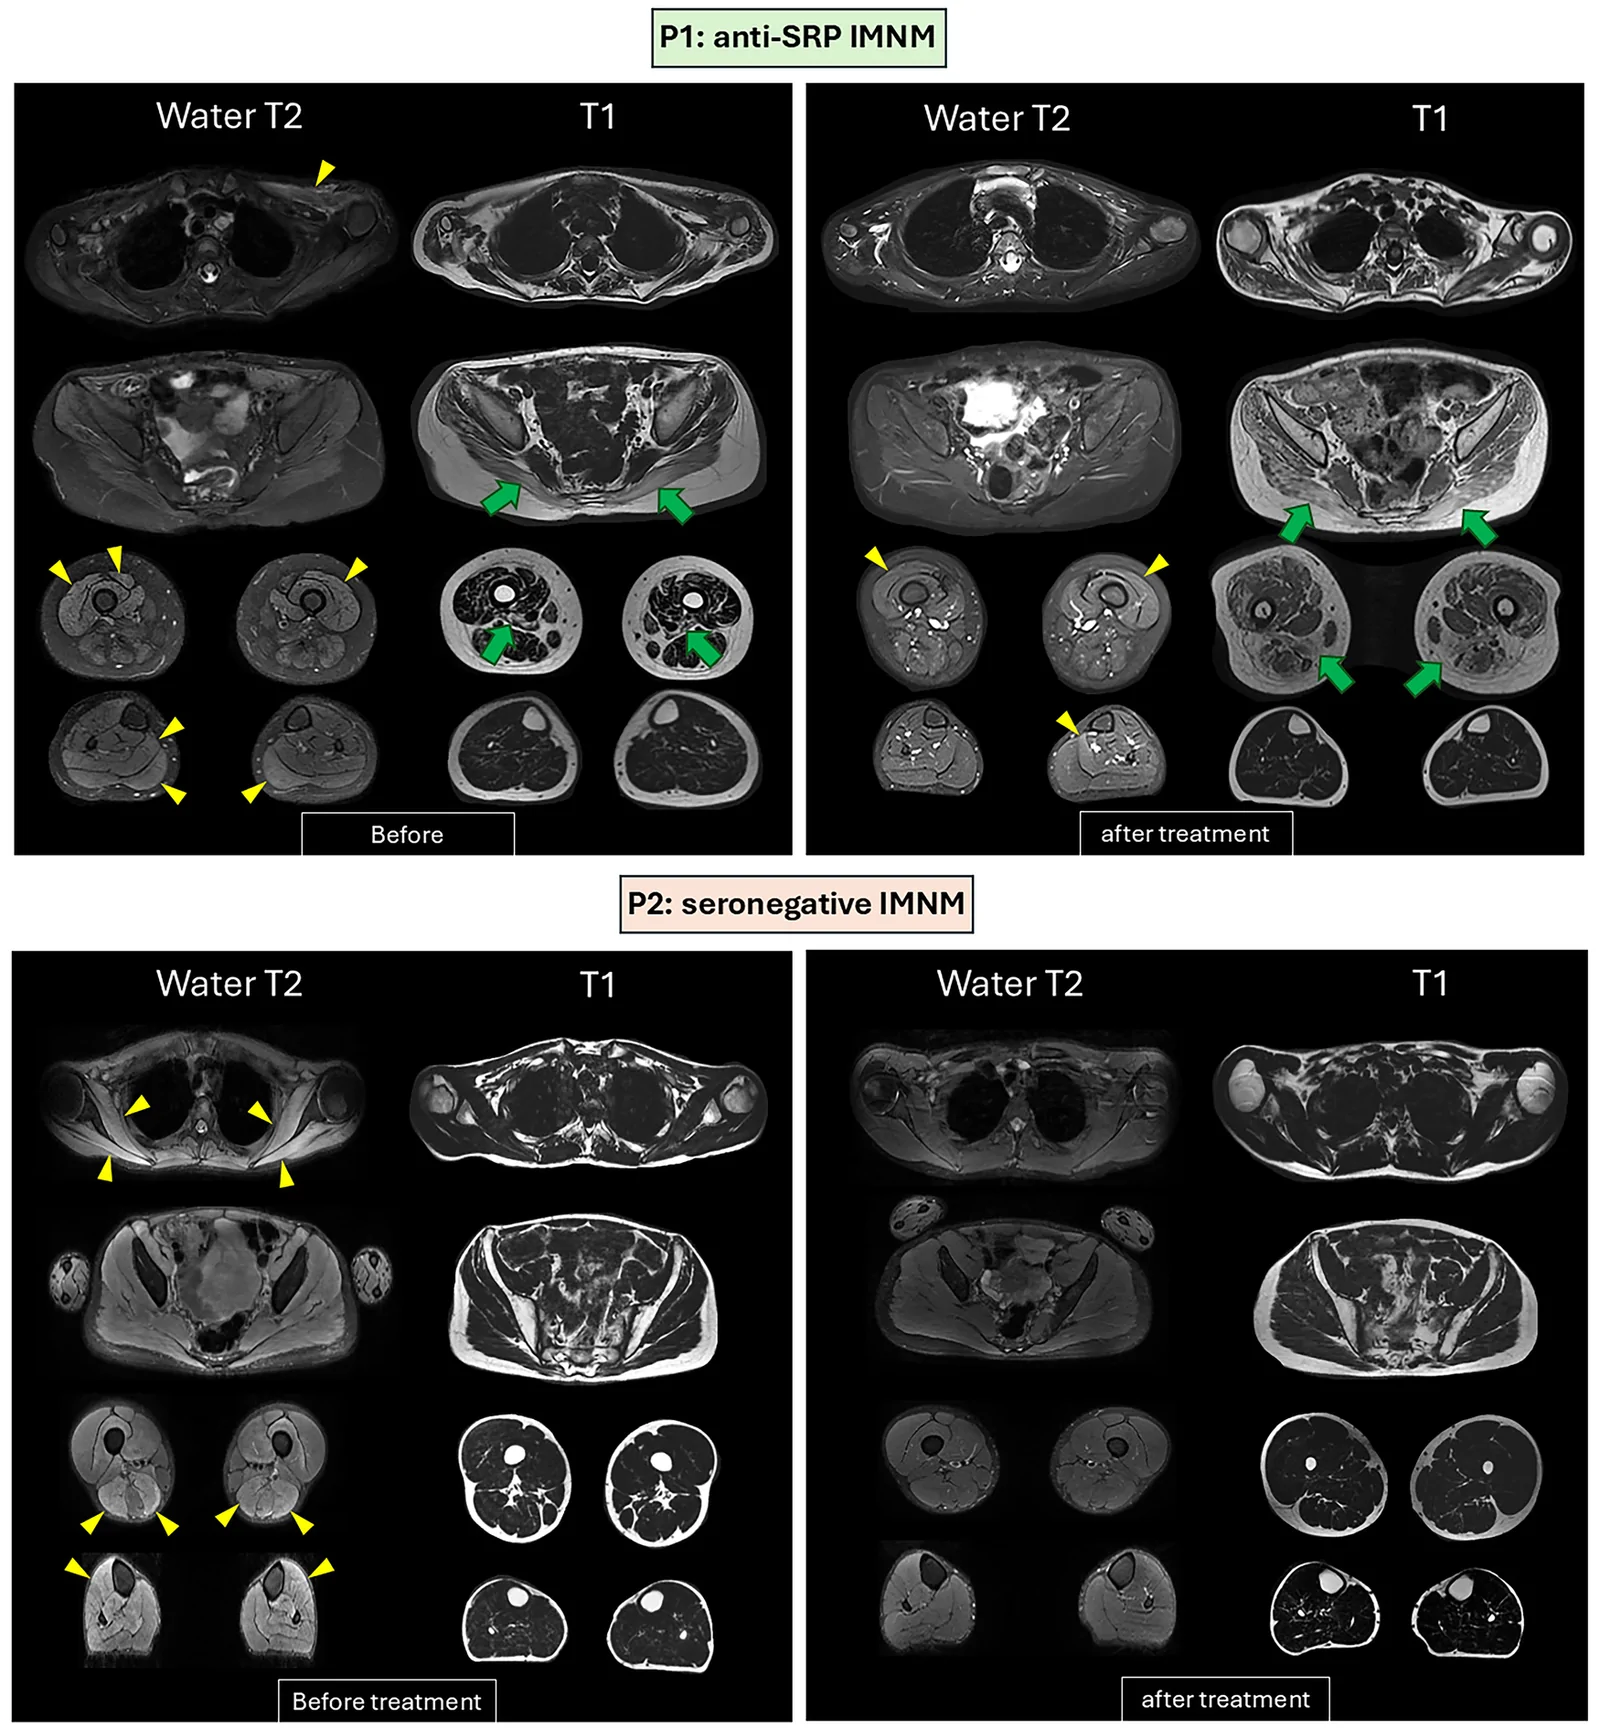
Muscle imaging features. Whole-body muscle MRI (axial T1-weighted and water-T2 sequences). P1 (anti-SRP IMNM): Before treatment, images show intense water-T2 muscle hypersignal (yellow arrowhead) and variable fibro-fatty replacement on T1-weighted sequences, especially in the bilateral *gluteus maximus* and *adductor magnus* muscles (green arrows). After treatment, there is persistence of water-T2 hypersignal in the lower limbs (yellow arrowhead) and worsening of fibro-fatty replacement on T1-weighted sequences (green arrows). P2 (seronegative IMNM): before treatment, images show diffuse water-T2 hypersignal, prominent in the periscapular muscles, posterior thigh compartment, and anterior leg compartment (yellow arrowhead), with no alterations on T1-weighted sequences. After treatment, there is global regression of the muscle hypersignal.
